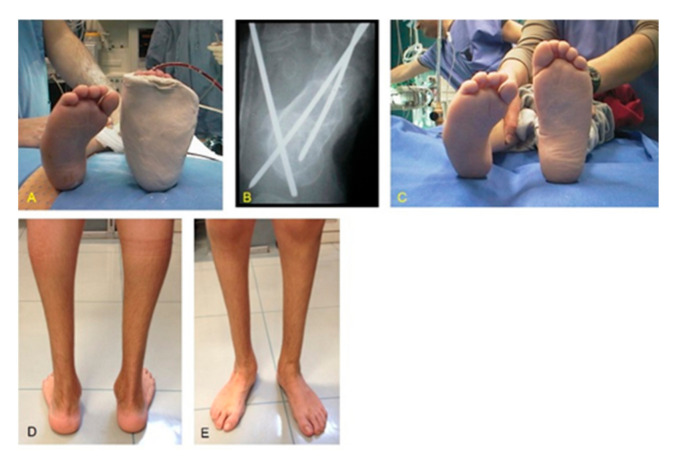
Same patient as shown in Figure 1: (A) postoperative cast immobilization after relapse surgery (ATL, PR, PM, tibialis anterior transfer, and cuboid osteotomy); (B) X-ray showing correction of the talocalcaneal relationship and K-wires fixation; (C) clinical aspect of both feet at the time of relapse surgery of CF right; (D) clinical results at a 18.2-year of follow-up with good alignment and correction of the hind foot; (E) frontal view showing correction of all CF components.
